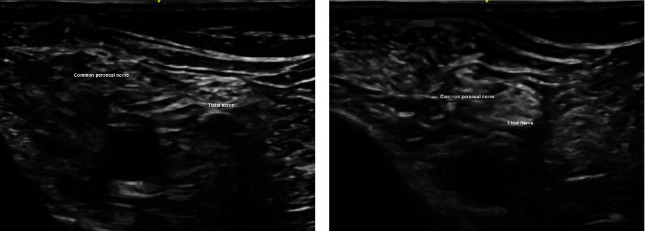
(a, b) Common peroneal nerve and tibial nerve converged as ultrasound probe, moving from caudal to cranial direction.
